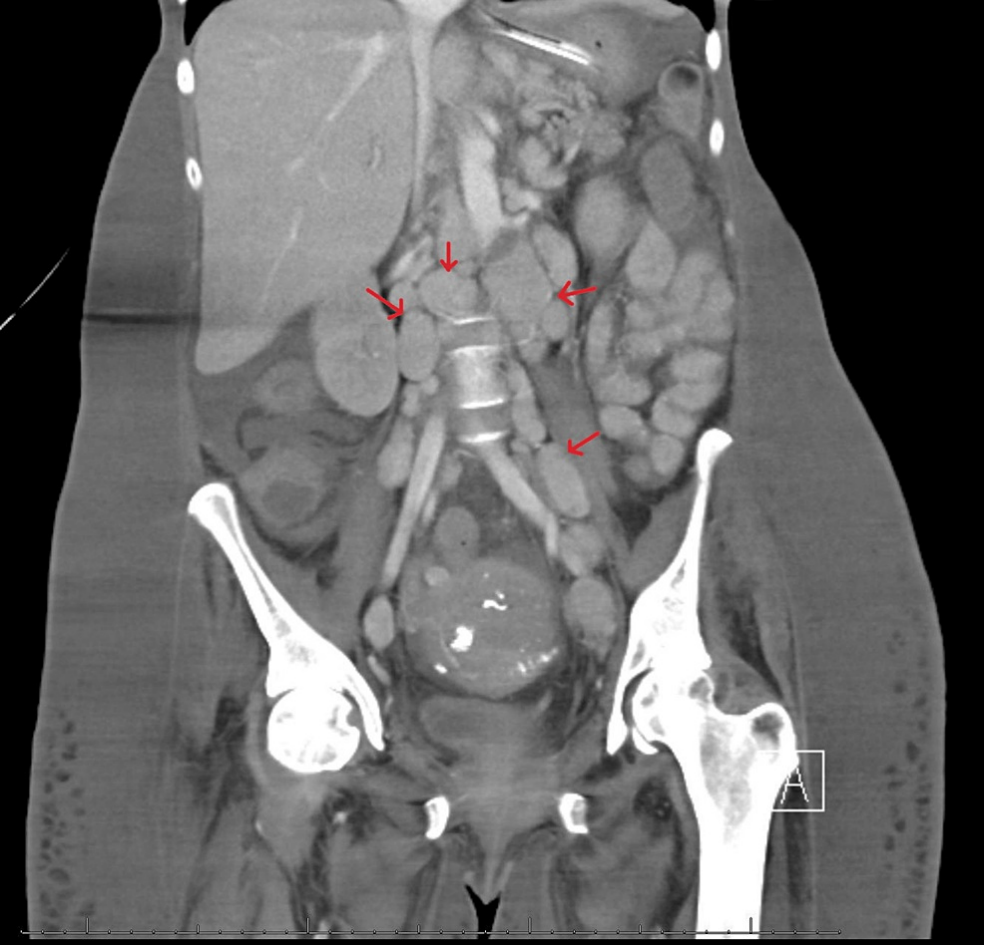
Computed tomography of the abdomen (coronal view) showing multiple enlarged retroperitoneal lymph nodes (red arrows).
